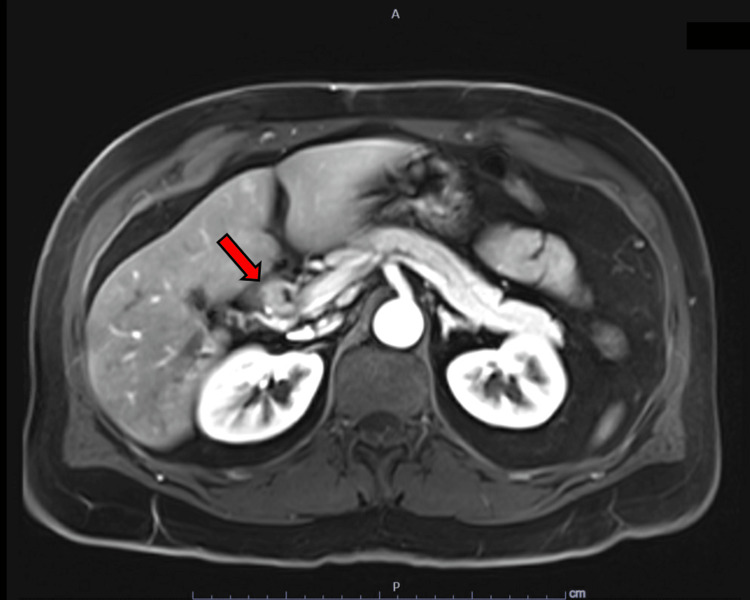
MRI arterial post-contrast demonstrates biliary mass Soft tissue (red arrow) extends over a length of greater than 4.2 cm and measures up to 1.8 cm in width. There is an additional enhancing soft tissue within the common bile duct at the bifurcation and extends to the right intrahepatic bile duct.
